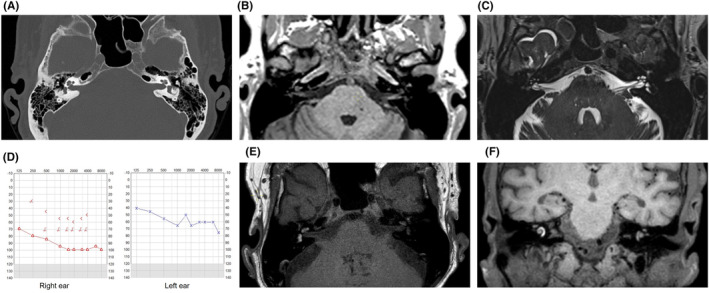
(A) CT scan of the ear without any inflammatory or neoplastic changes in the middle ear and normal otic capsule. (B) Pre‐contrast axial T1‐weighted MRI imaging showing a slight hyperintensity in the area of the cochlea/vestibular apparatus on the right side and in the cochlea on the left side due to the presence of methemoglobin from blood. (C) T2‐weighted MRI imaging showing loss of perilymph signal mainly in the scala tympani and in the vestibule in the right cochlea. (D) Tone audiogram on day 7. (E, F) Follow up pre‐contrast axial T1‐weighted MRI and fat saturated coronal images showing even more distinct signs of bilateral inner ear hemorrhage.
